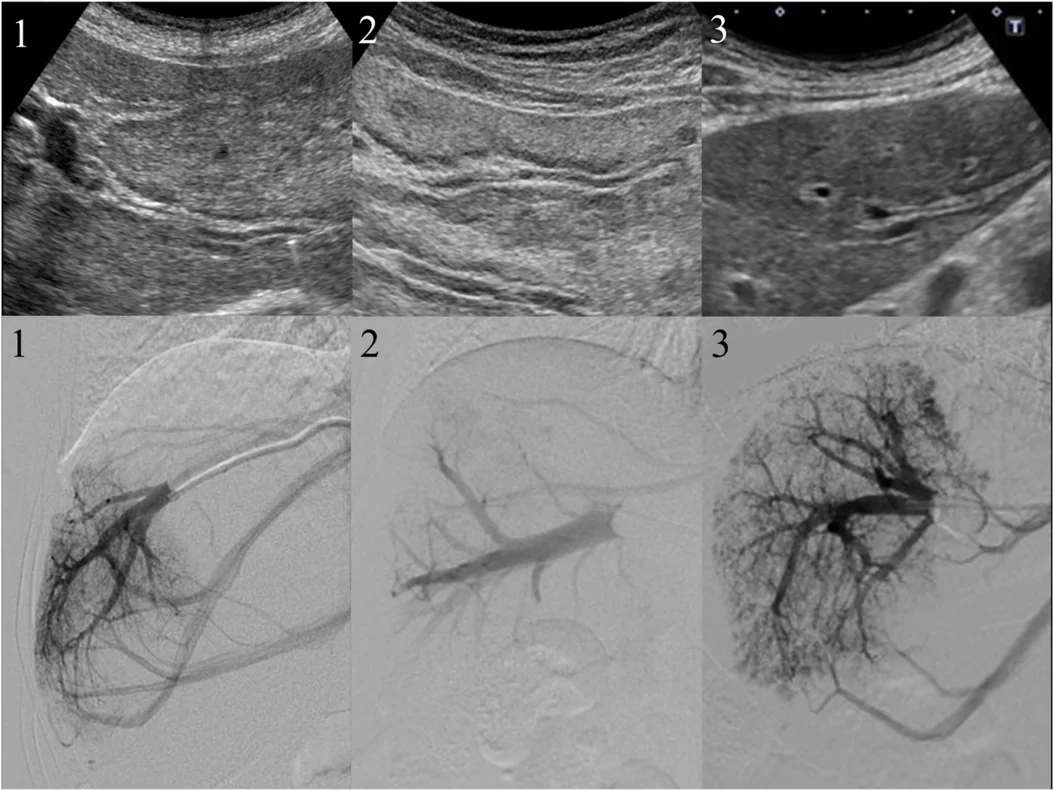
Upper: liver ultrasonography. The examinations revealed the “portal sandwich sign”. Lower: Hepatic venography. Hepatic venography shows anastomosis between hepatic veins. Case 1 is reproduced from Ando Y et al. Journal of Medical Case Reports. 2025;19:392, under CC BY‐NC‐ND 4.0.
